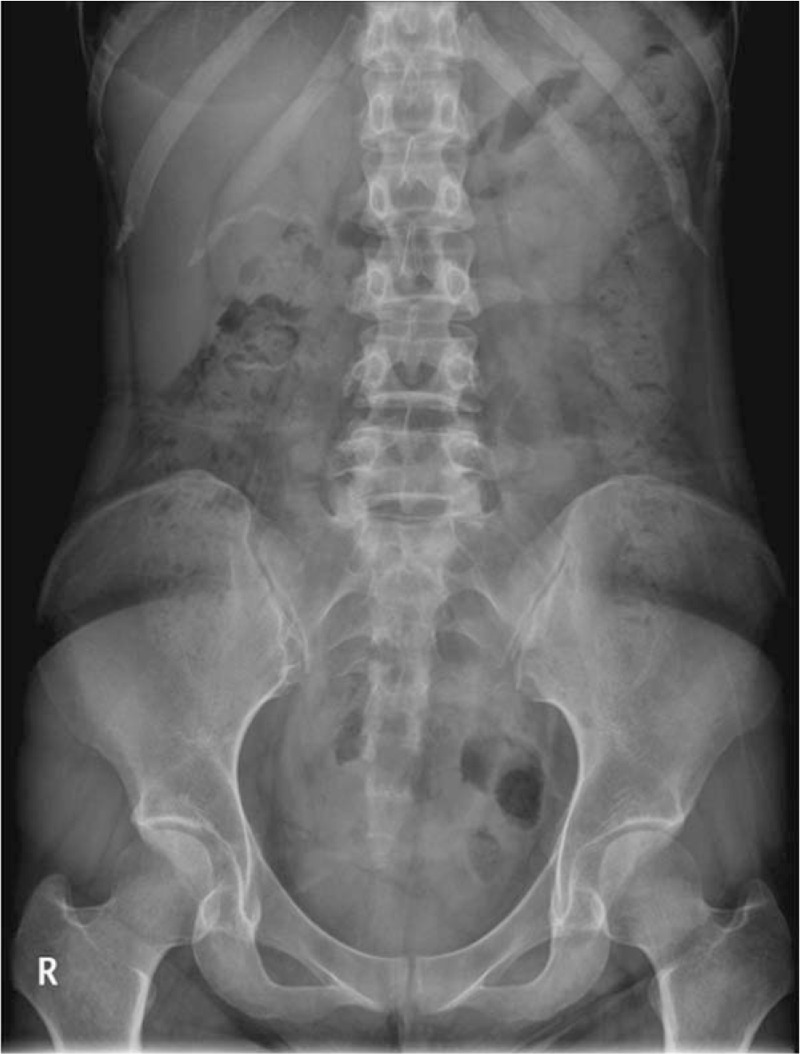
Radiograph 12 months after capsule endoscopy shows disappearance of the capsule.
